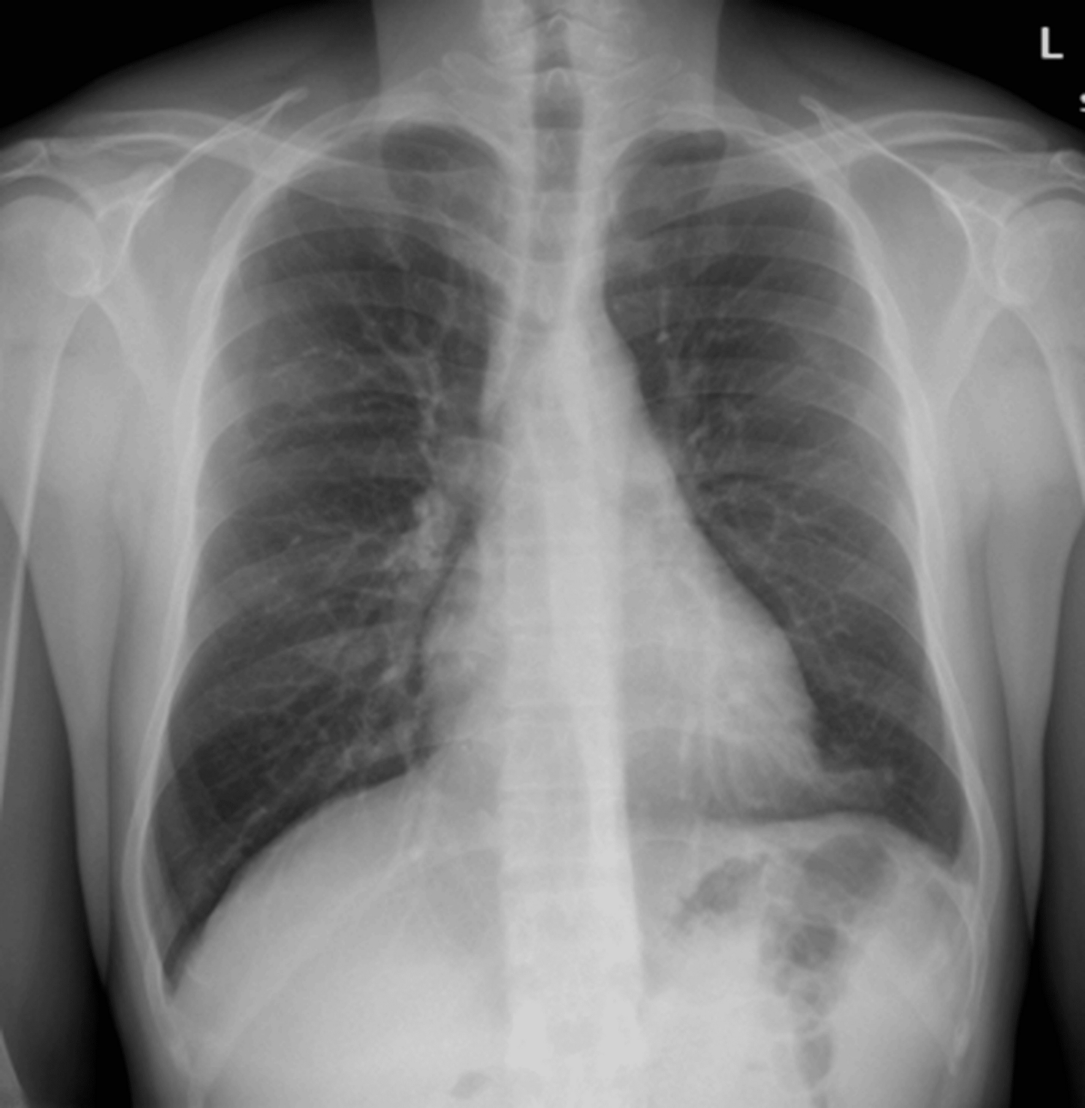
Chest X-ray (PA view), normal findings. PA: posterior anterior.
